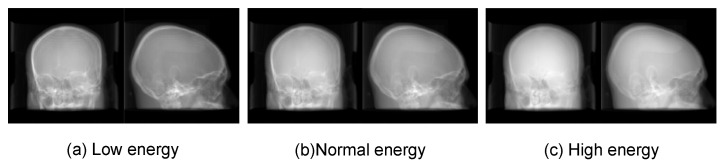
Examples of CT-DRR datasets in different X-ray energies.
